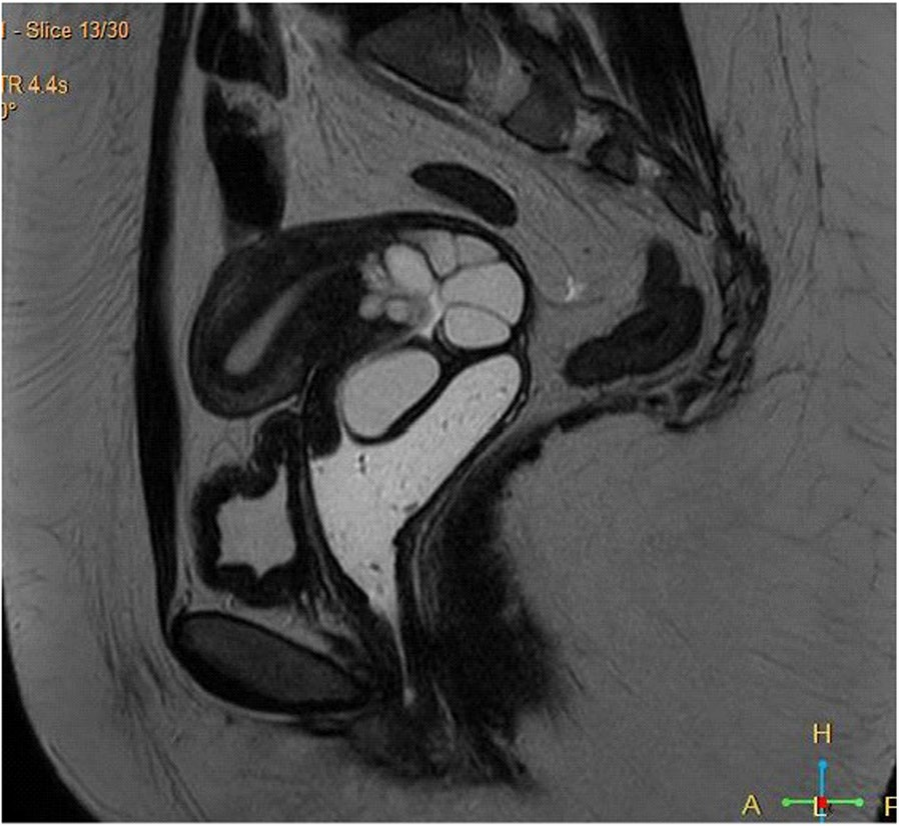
Magnetic resonance imaging: sagittal T2- weighted image showing large, hyperintense cervical cysts
